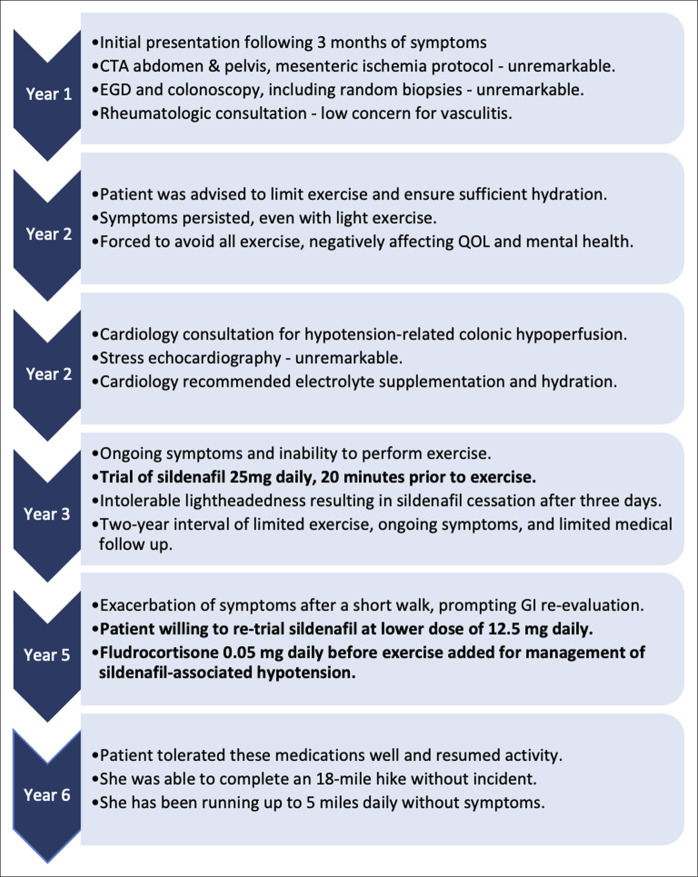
Summary of case events. CTA, computed tomography angiography; EGD, esophagogastroduodenoscopy; GI, gastrointestinal; QOL, quality of life.
